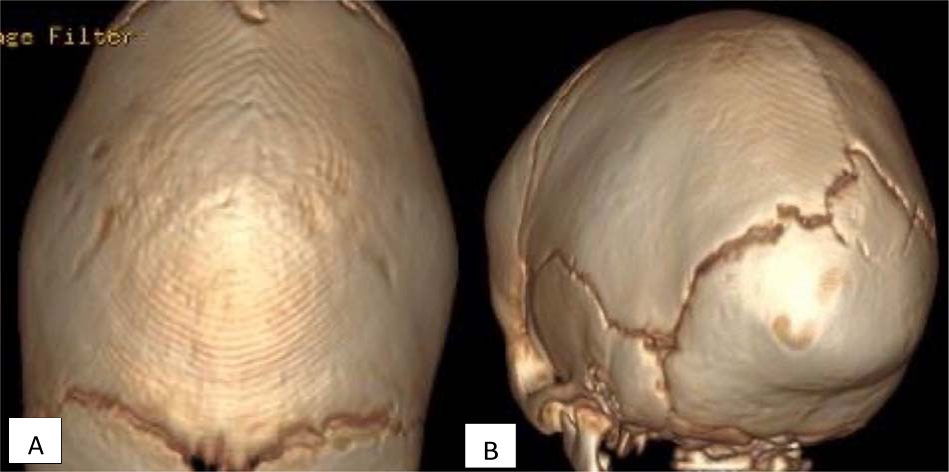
Initial computed tomography scan (CT) scan obtained when the patient was 3 months old showing sagittal craniosynostosis. (A) Superior axial view and (B) posterior view.
